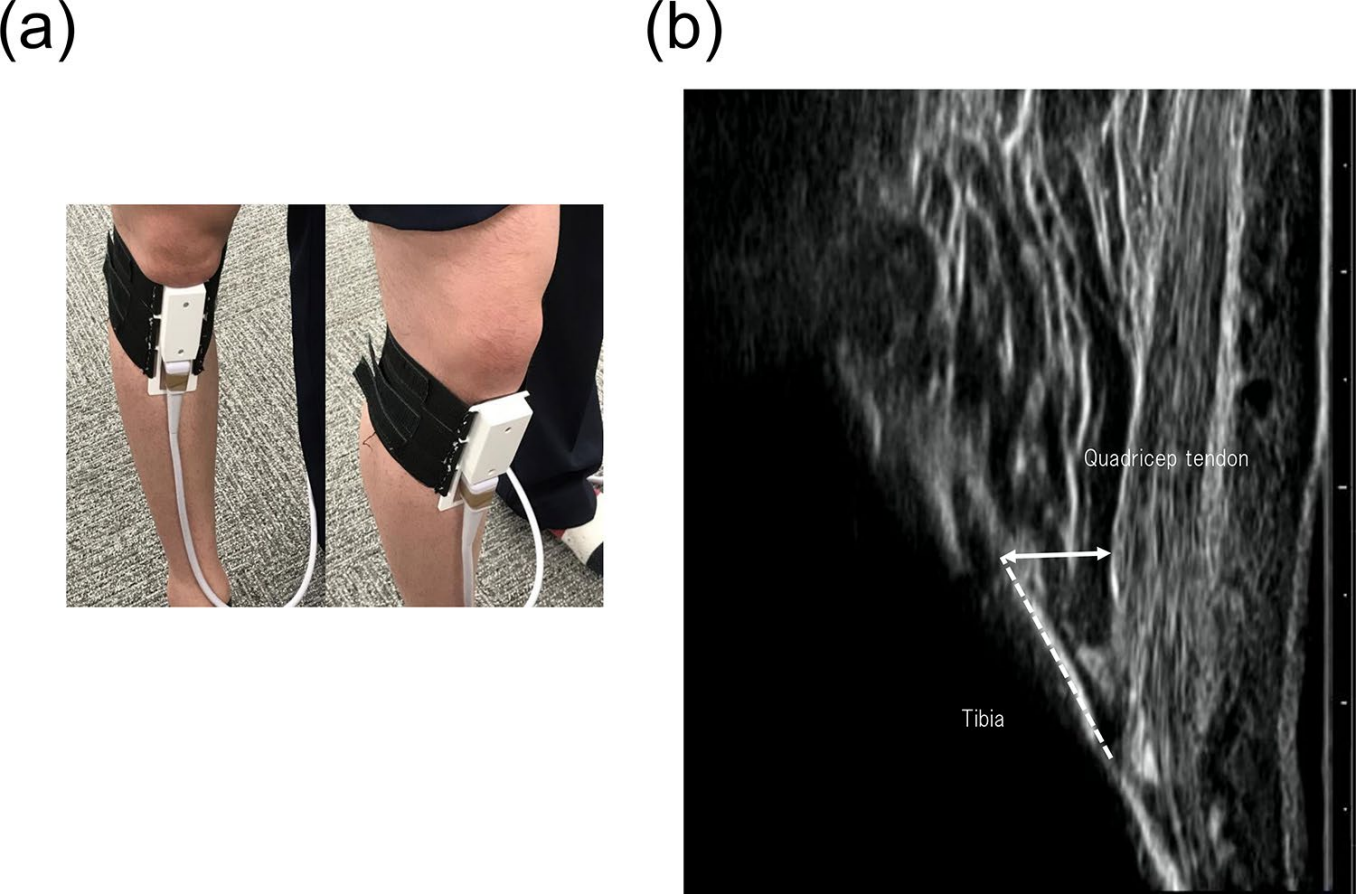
Imaging of the infrapatellar fat pad and definition Transducer placement (a) and representative ultrasound images (b). The dotted line shows the reference line on the tibia, which is 10 mm along the origin of tenderness in the quadriceps on the proximal tibia. The double arrow indicates the thickness of the IFP and perpendicular distance between the proximal tibial line and patellar tendon. IFP, infrapatellar fat pad
